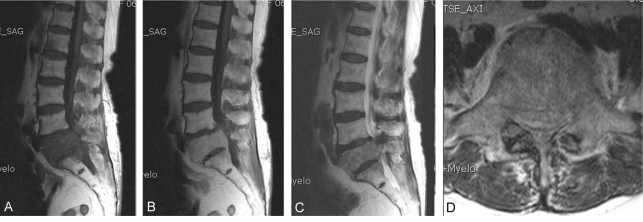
(A) A sagittal T1-weighted magnetic resonance imaging scan shows low signal bone marrow infiltrations involving L1, 5 and S1 vertebral bodies. (B) On enhances imaging, the lesions were homogenously enhanced. (C) A sagittal T2-weighted scan shows isosignal intensity at the same lesion and preservation of intervertebral disc. There was no evidence of paravertebral abscess. (D) Axial scan shows the stenotic condition resulting from epidural mass and facet arthritis. There was no evidence of paravertebral soft tissue abnormalities.
